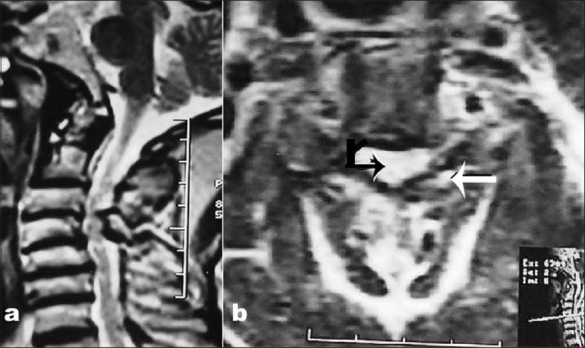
T2-weighted sagittal (a) MRI showing an OLF opposite the C3–4 vertebral bodies. There is associated cervical kyphosis with decreased disc space at the C3–4, C4–5 and C5-6 levels with significant spinal canal compromise and hyperintense cord changes. The axial weighted images (b) reveal a left-sided laterally situated, linear OLF (horizontal straight arrow) causing thecal compression (curved arrow)
